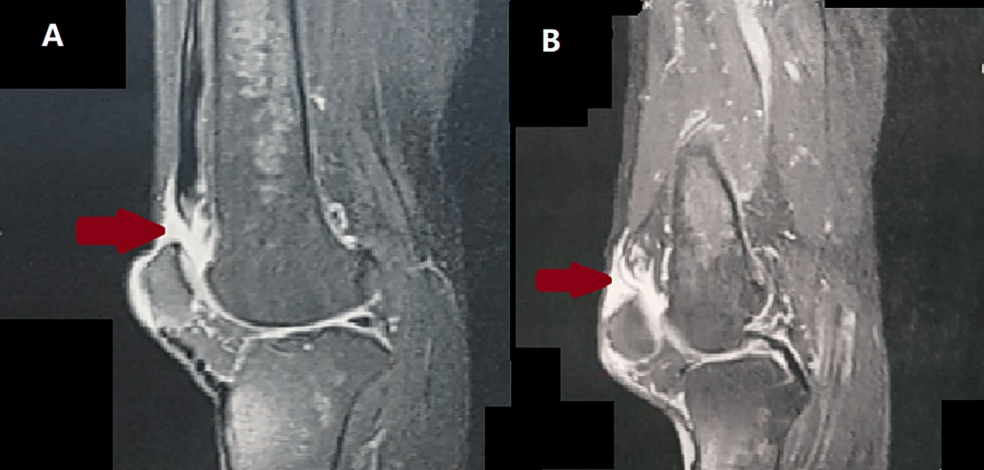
MRI sagittal section of the right knee (A) and left knee (B) showing a rupture of both quadriceps tendons.
